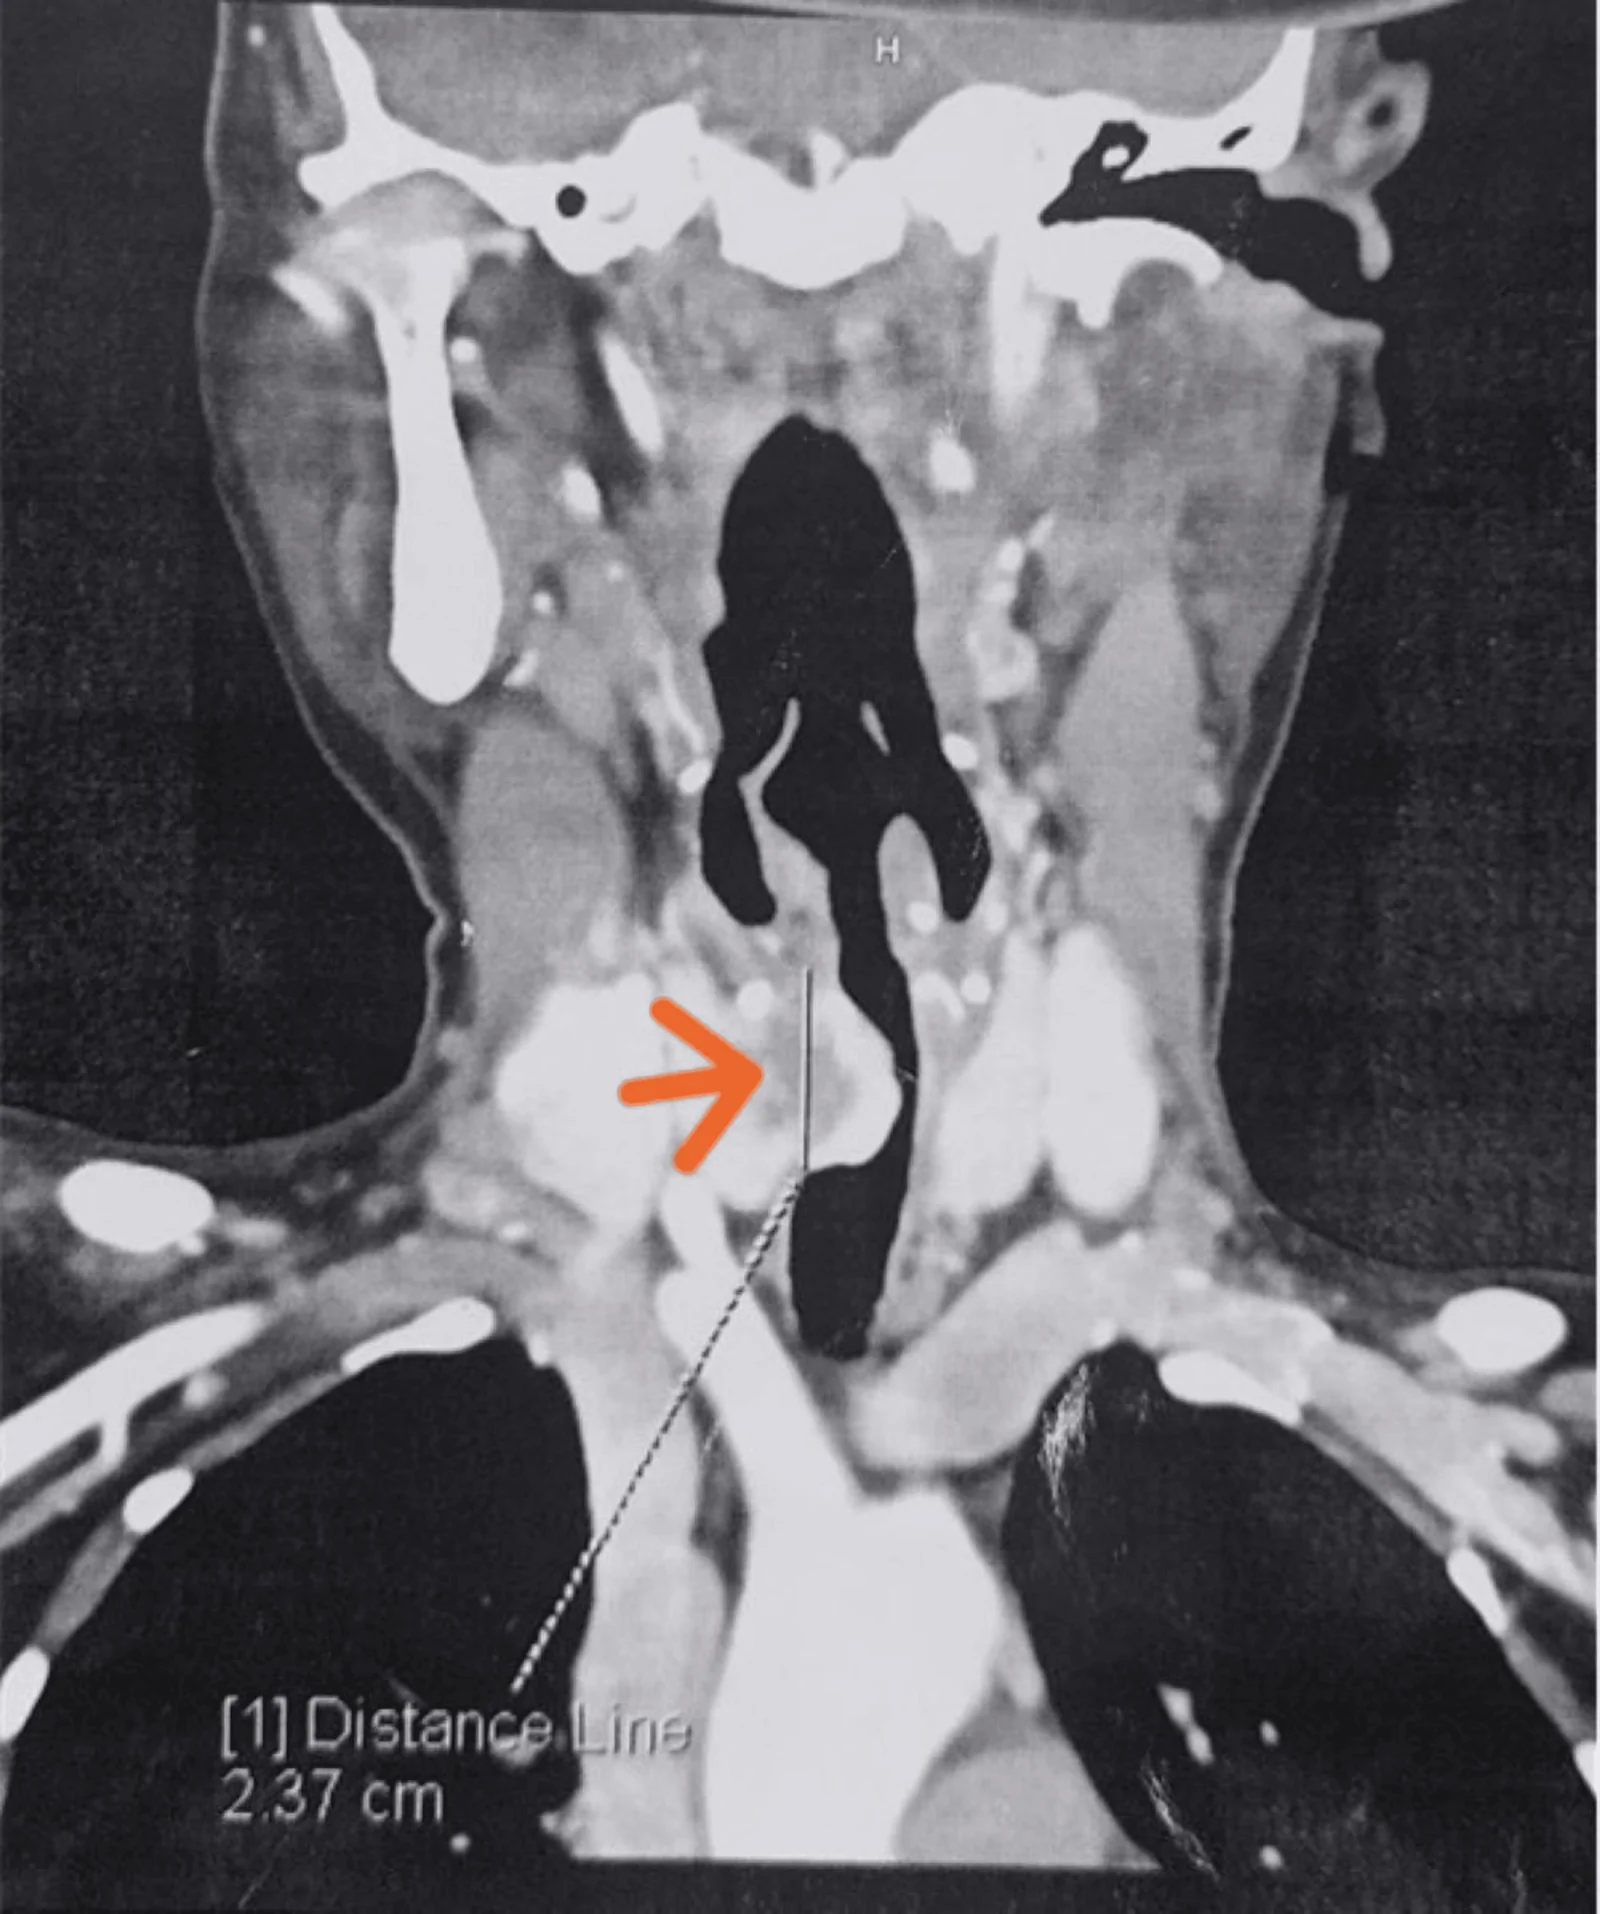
Contrast-enhanced computed tomography of the neck (coronal view) demonstrating a tumor infiltrating the larynx and trachea (orange arrow).
